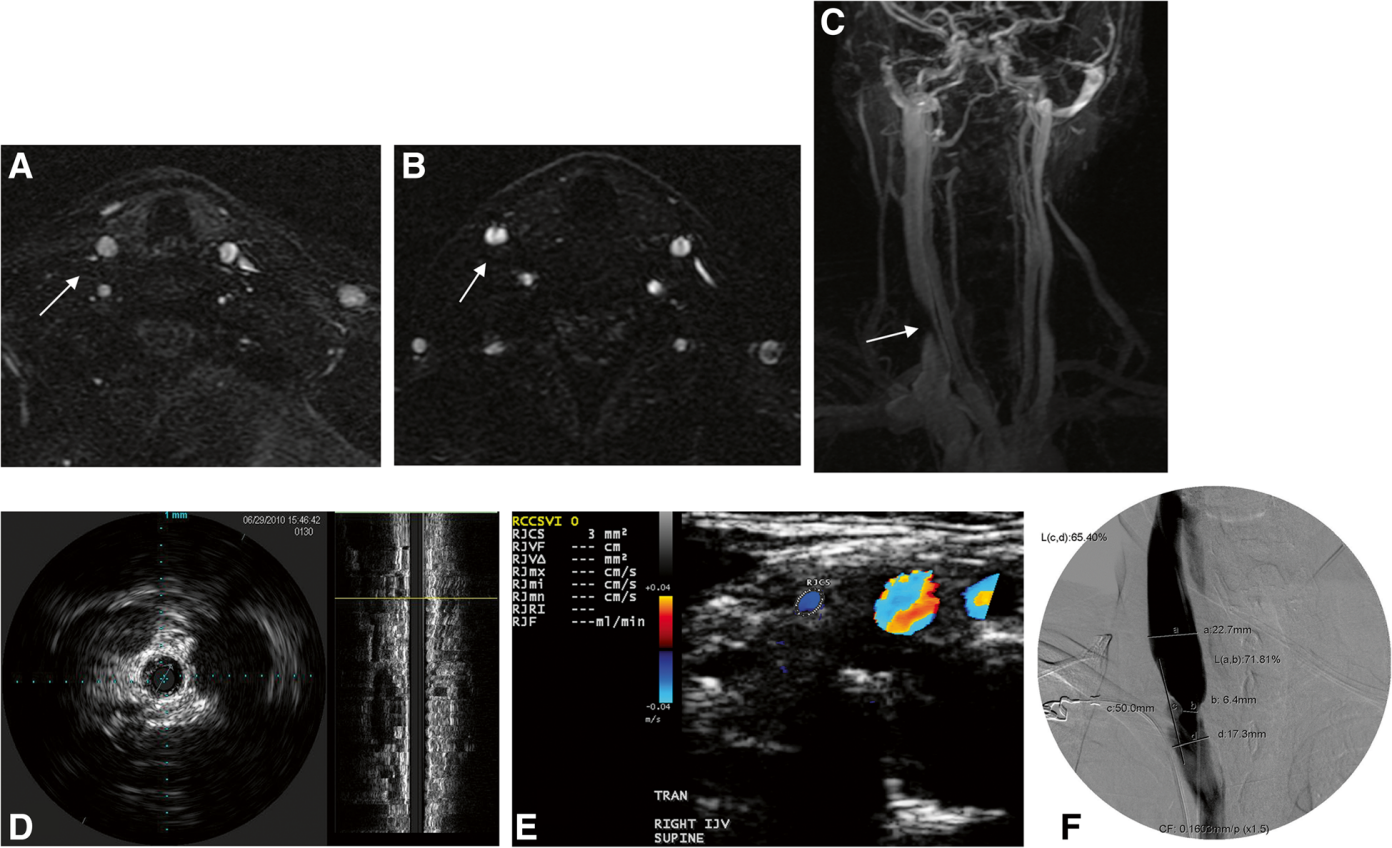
Multimodal imaging approach of extracranial neck veins. Axial 2D time-of-flight (A), enhanced 3D-time resolved imaging of contrast kinetics (B &C), intravascular sonography (D), Doppler sonography (E), and catheter venography (F) all showing venous abnormality of the right internal jugular vein (significant narrowing-arrows) in the same patient.
